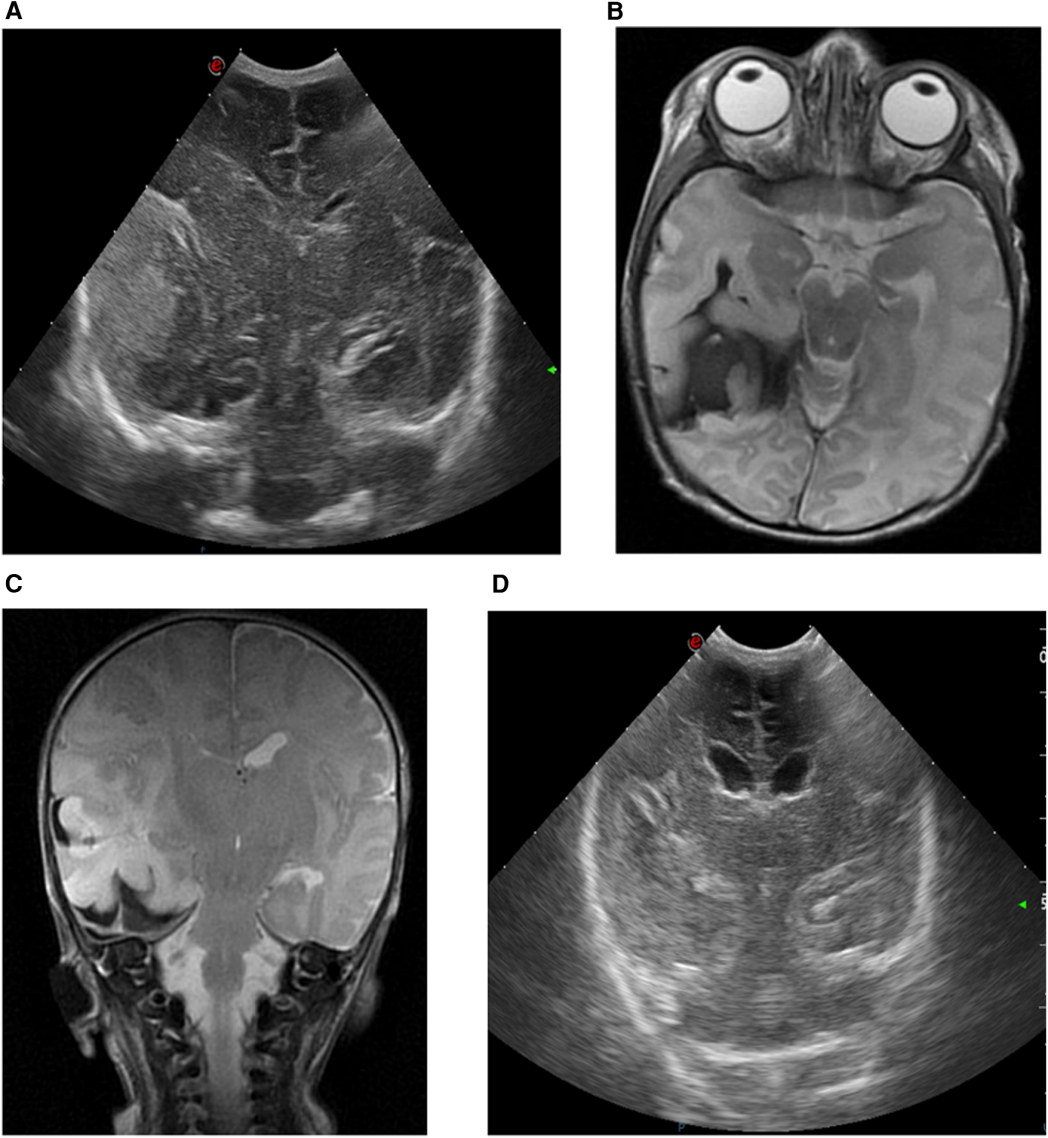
cUS and MRI brain findings of case 3. (A) cUS coronal view, showing a right-sided echogenic extra-axial lesion, presumably subdural and temporally located, with midline shift. (B) MRI T2-weighted sequence, axial view, and (C) MRI T2-weighted sequence, coronal view, showing a large extra-axial hemorrhage in the temporal region on the right side with a maximal thickness of 16 mm and a midline shift of 7 mm to the left. The combined diameter of the subdural hematoma and intraparenchymal hemorrhage is 29 mm. (D) cUS coronal view, after surgical intervention.
